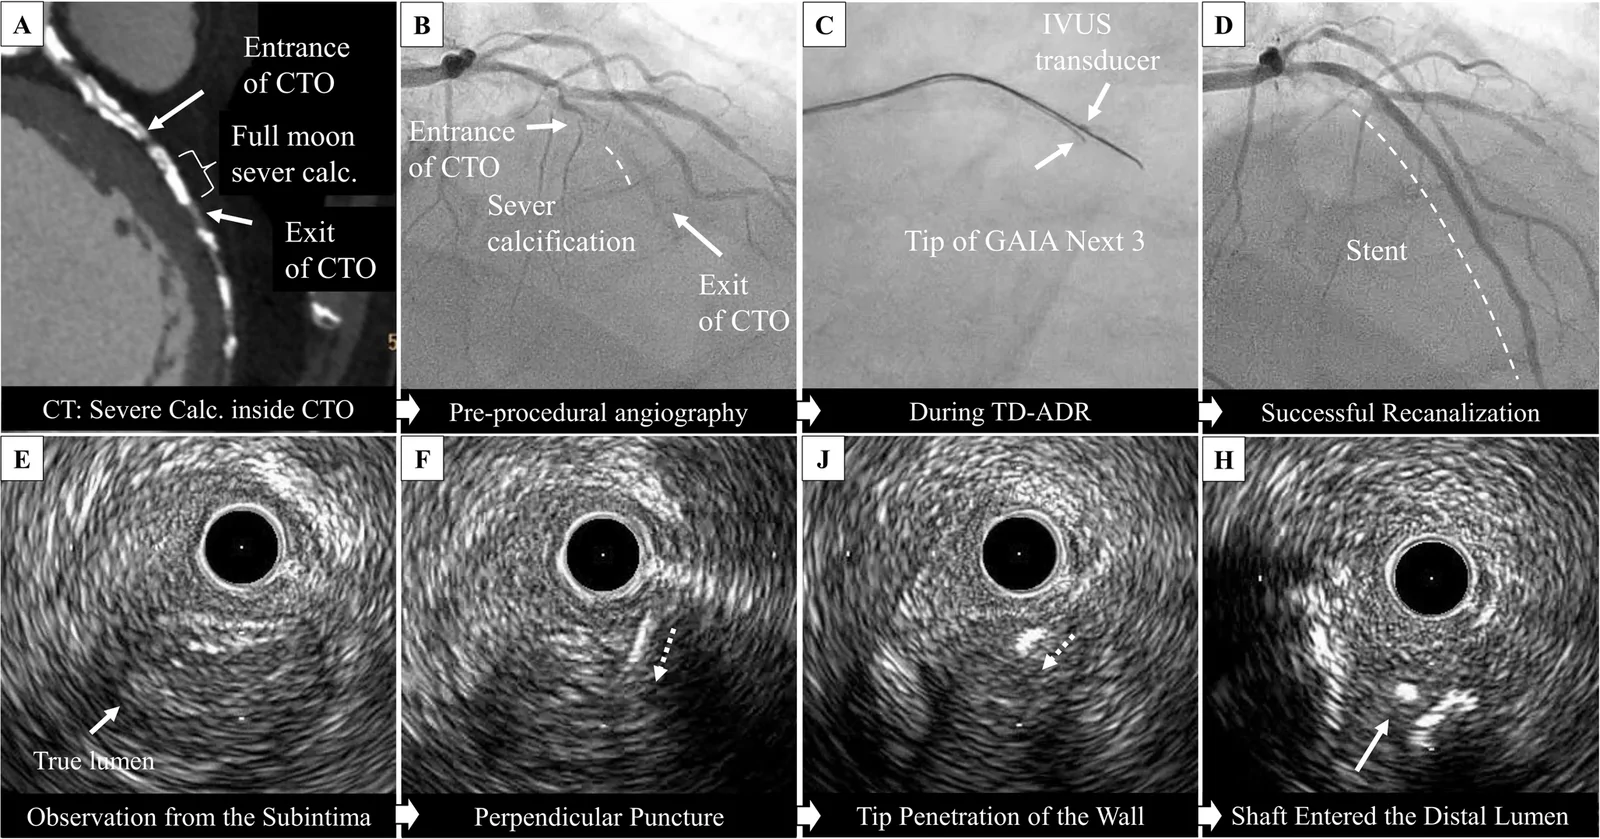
First TD-ADR Case. Computed tomographic image (A), angiographic images (B) prior to, C during, and D after the procedure, and intravascular ultrasound images E prior to, F, G during the puncture, and H after successful puncture. *CTO* chronic total occlusion, *IVUS* intravascular ultrasound, *TD-ADR* tip detection-antegrade dissection re-entry.
